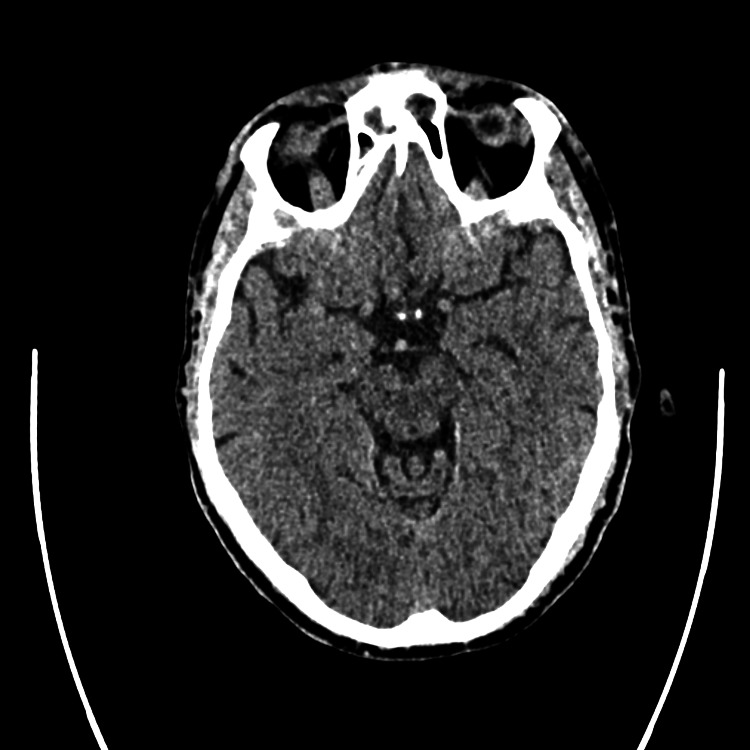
Normal computed tomography of the head without evidence of intracranial hemorrhage.
